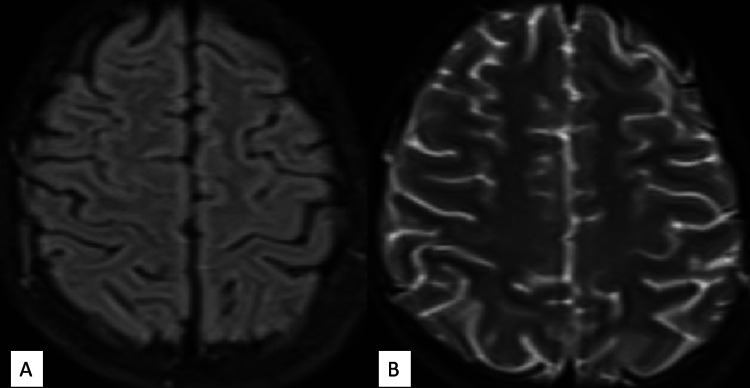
(A&B): Follow-up MRI showing the disappearance of oedema, characteristic of posterior reversible encephalopathy syndrome.
